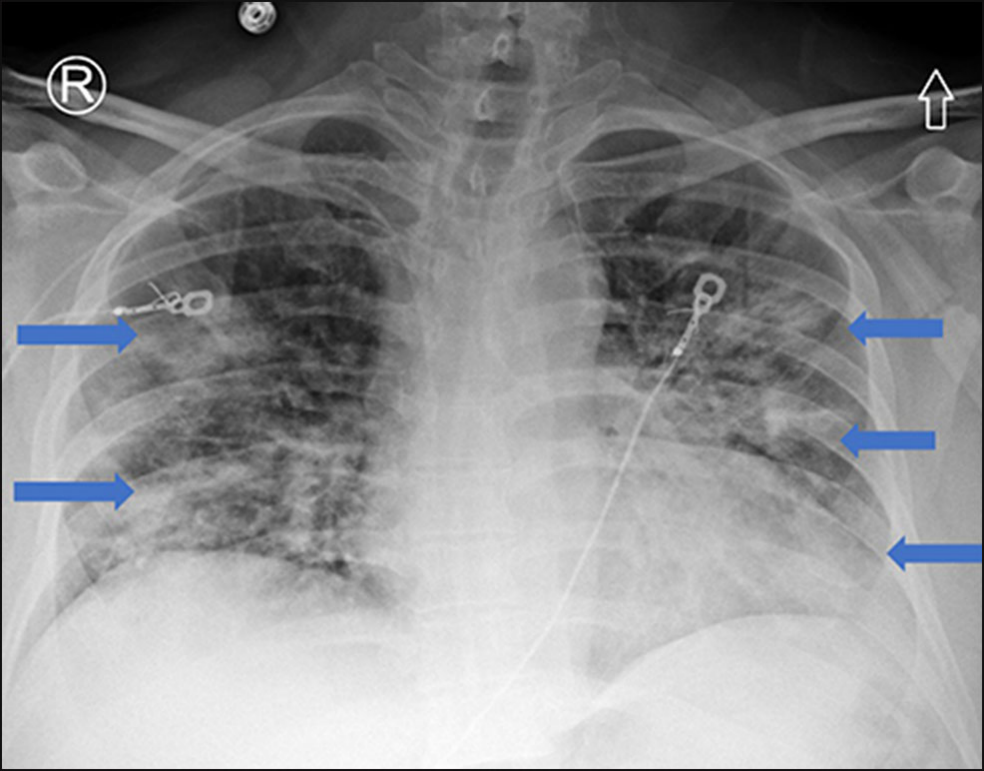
Chest X-ray findings of COVID-19 pneumonia: frontal radiograph of the chest demonstrates low lung volumes with bilateral perihilar ground glass opacities and peripheral airspace consolidations (blue arrows) in a predominately mid and lower lobe distribution.
